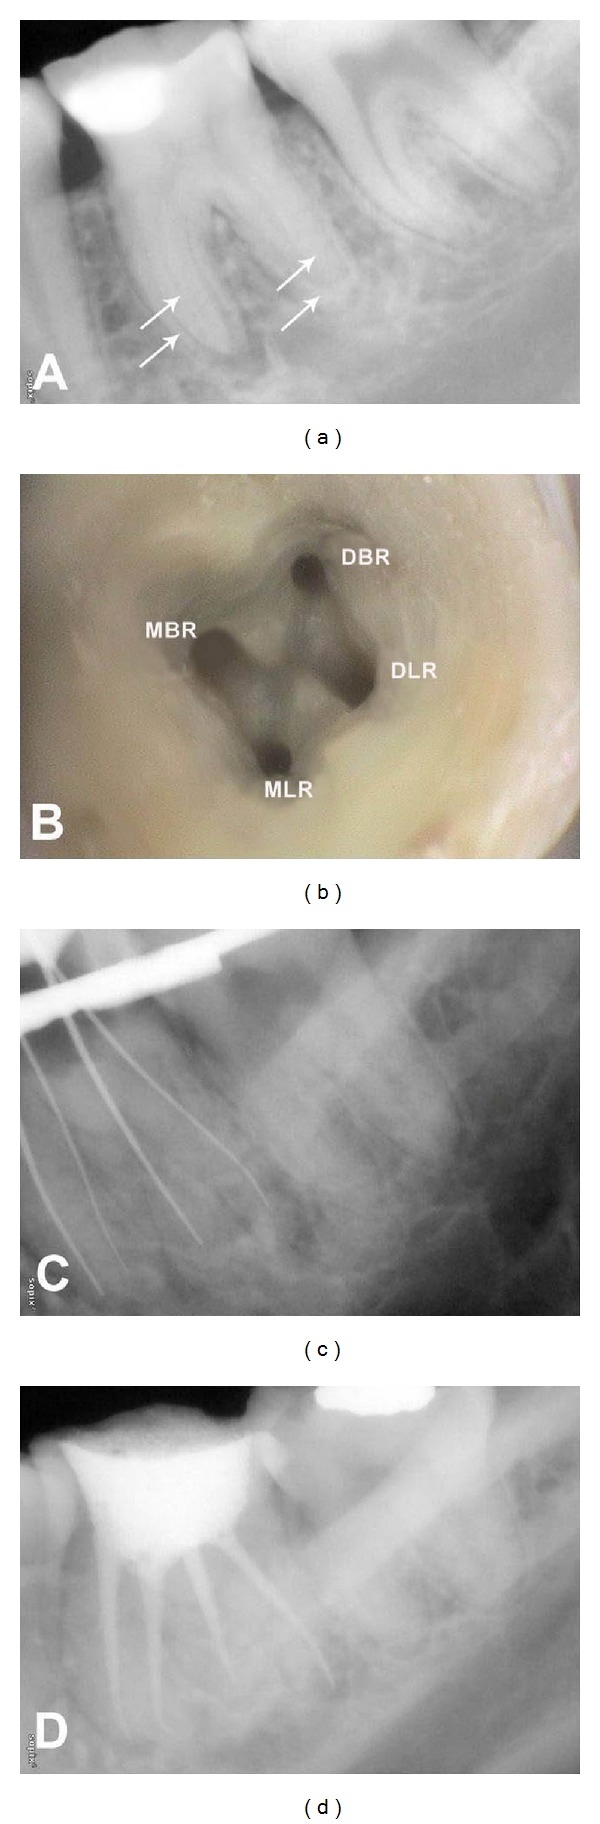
(a) Preoperative radiograph of tooth 19. (b) Access opening showing four canals (MBR: Mesiobuccal; MLR: Mesiolingual; DBR: Distobuccal; DLR: Distolingual). (c) Working length radiograph of tooth 19 in eccentric angulation. (d) Postobturation radiograph.
